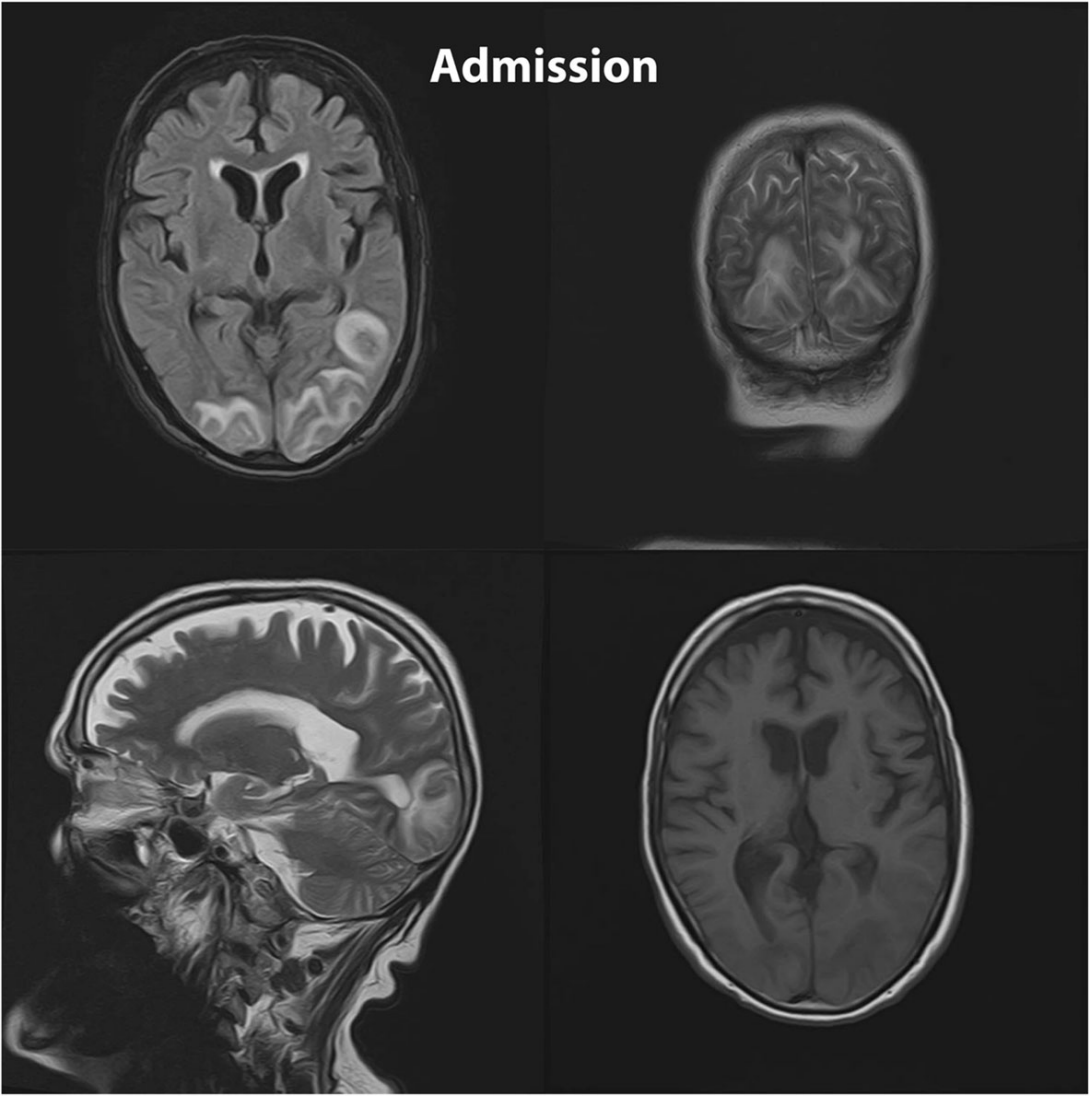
(Case 2): Admission MRI: MRI shows symmetric involvement of the parasagittal areas of bilateral parietal and occipital lobes. Asymmetric involvement of bilateral posterior temporal lobes is also evident. The affected areas are gray matter, gray white matter junction, and subcortical white matter. The lesions signal on T2-weighted and FLAIR sequences are high and low on T1-weighted sequence. No evidence of Diffusion restriction is depicted in involved areas. This patient refused follow-up MRI after chelation therapy as he believed to be completely fine
